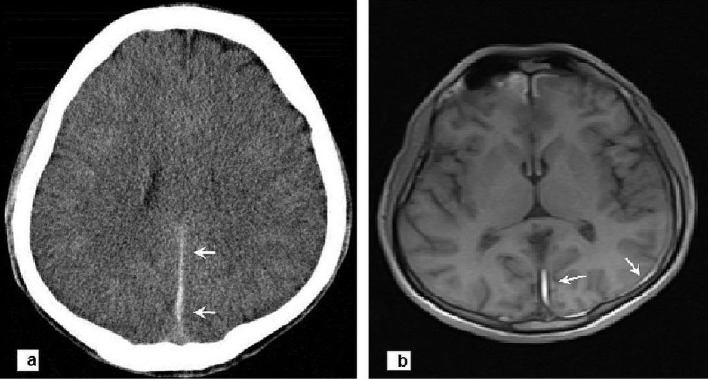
(a) Axial CT scan of brain performed 4 h after the accident revealing a small left-sided acute interhemispheric subdural hematoma, which was posteriorly located (arrows). (b) Cranial MRI showing minimal ASH in the posterior interhemispheric fissure (arrows) with subacute subdural hematoma in the left parieto-occipital cortex and occipital regions (arrows).
